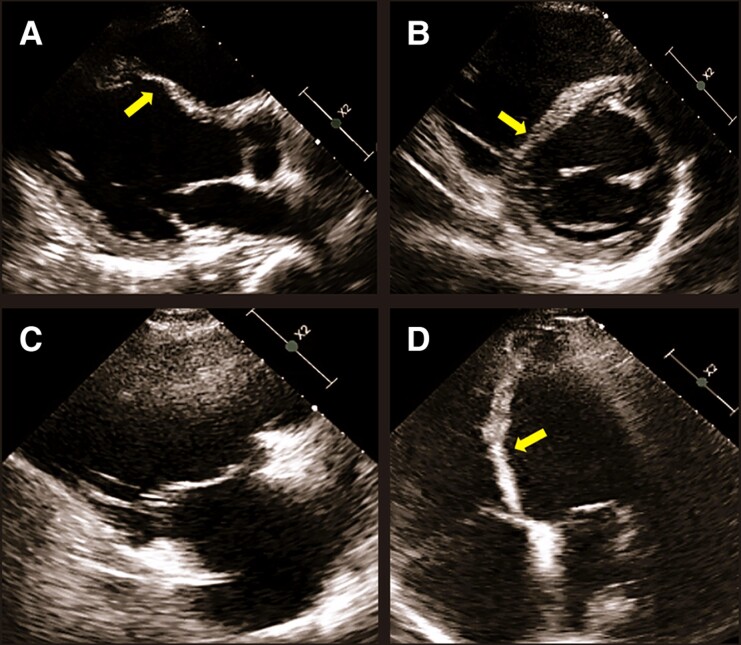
(Patient 1) Echocardiogram. Transthoracic echocardiography showing a moderately dilated and severely hypokinetic left ventricle (index end-diastolic volume 92 mL/m^2^, ejection fraction 27%) with thinned and dyskinetic mid-basal interventricular septum (*A, B*, and *D*, arrow). The right ventricle was severely dilated and akinetic, notably in the inferior wall (end-diastolic area 36 cm^2^ and fractional area change 13%) (*C*).
